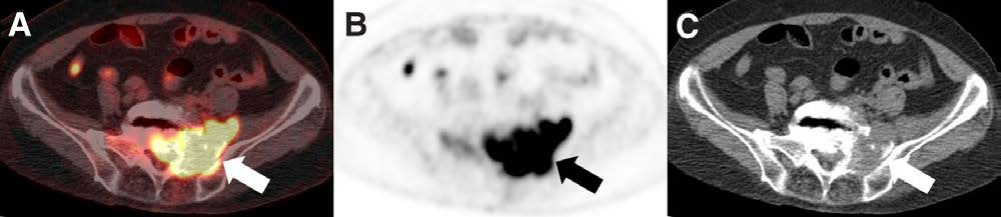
A 78-year-old woman who had sacral diffuse large B cell lymphoma (DLBL). Axial (A) PET/CT, (B) PET, and (C) CT images show a 6.7 cm mass in the sacrum with a visual score of 4 and homogenous uptake. The tumor-to-liver ratio (TLR), maximum standardized uptake value (SUVmax), and total lesion glycolysis (TLG) were 15.1, 33.7, and 401.2, respectively. The mass was proven as sacral DLBL after needle biopsy. DLBL = diffuse large B cell lymphoma, CT = computed tomography, PET = positron emission tomography, TLR = tumor-to-liver ratio.
